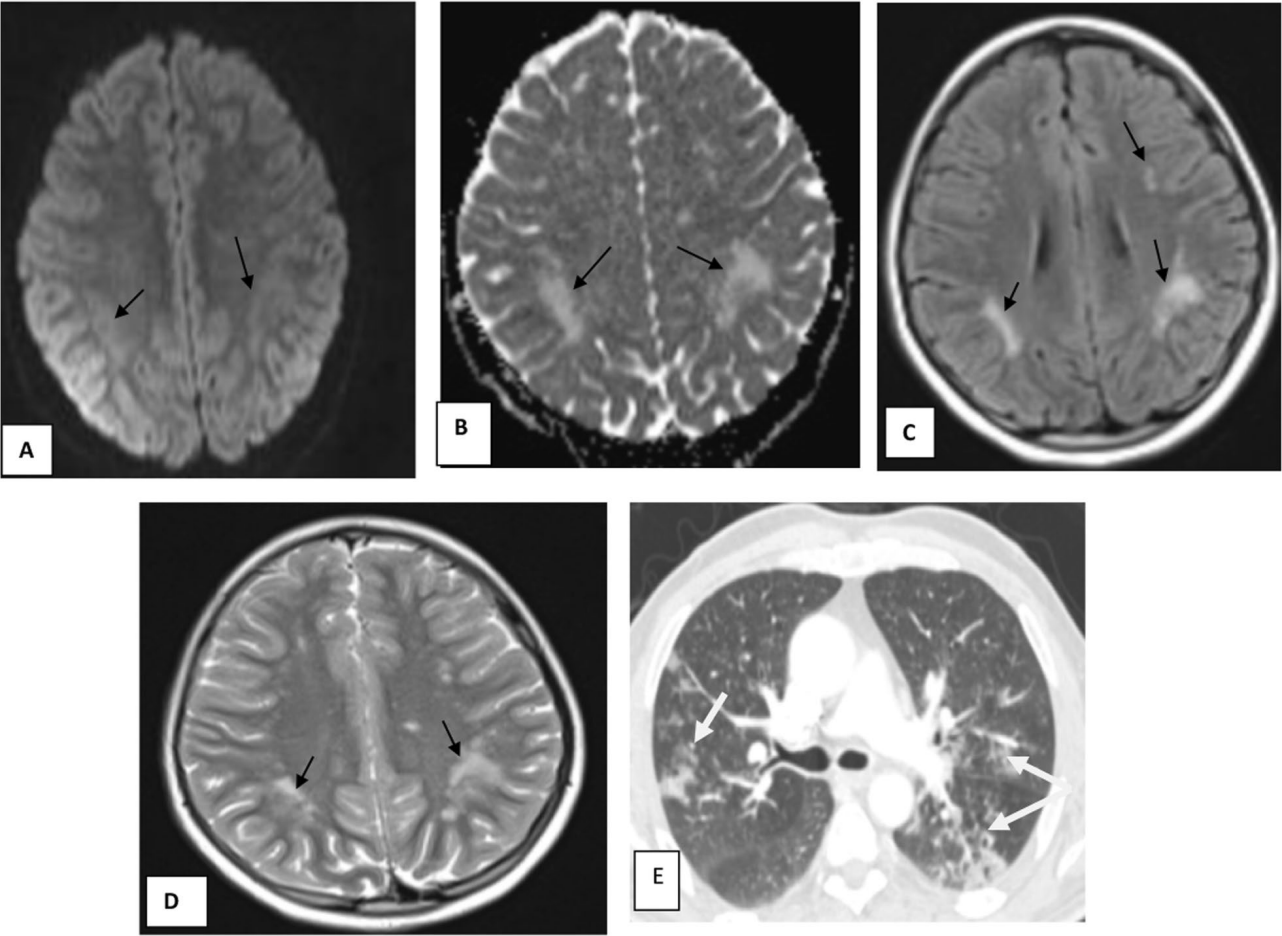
Male patient aged 28 years old, with history of COVID-19 infection. MRI of brain shows multiple patchy areas of abnormal signal intensity seen at subcortical white matter bilaterally (black arrows). They display high signal intensity in FLAIR and T2WI sequences (C, D), no diffusion restriction at DWI and ADC (A, B), no surrounding edema or mass effect. ADEM is considered in this case. Axial CT (E) chest cut of the same patient shows bilateral peripheral ground-glass opacities (white arrows) (moderate severity)
